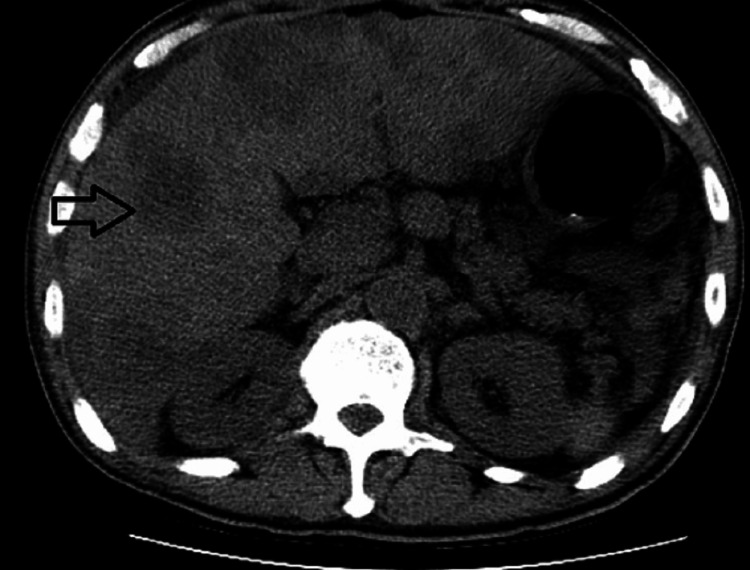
CT abdomen without contrast CT abdomen without contrast revealed hepatomegaly with multiple hypodense masses representing metastatic disease (black arrow).
